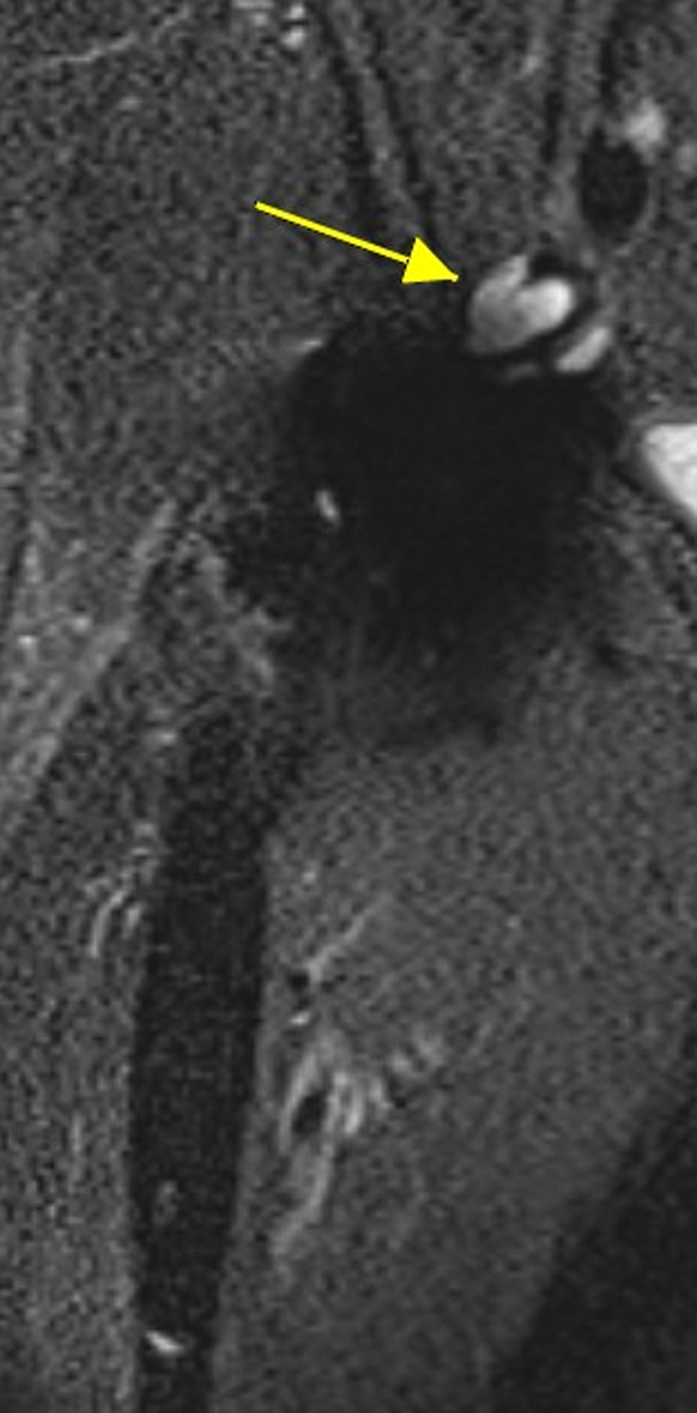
metal artifact reduction sequence MRI revealing fluid collection (yellow arrow) in the anterior aspect of the right hip extending into the iliopsoas bursa. Coronal short tau inversion recovery setting.
